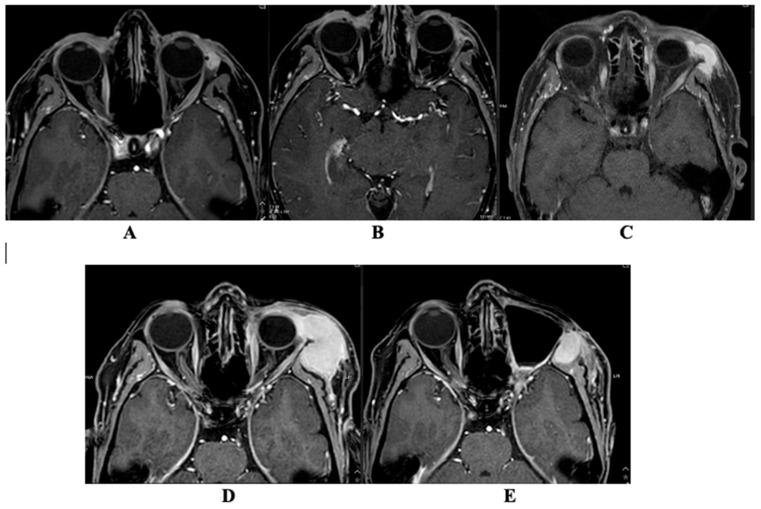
Magnetic resonance imaging (MRI) changes on an axial plane showing lesion-related changes in the left orbital region. (A) MRI performed 21 December 2022; (B) MRI performed 7 June 2023; (C) MRI performed 27 May 2024; (D) MRI performed 14 August 2024; (E) MRI performed 8 January 2025.
